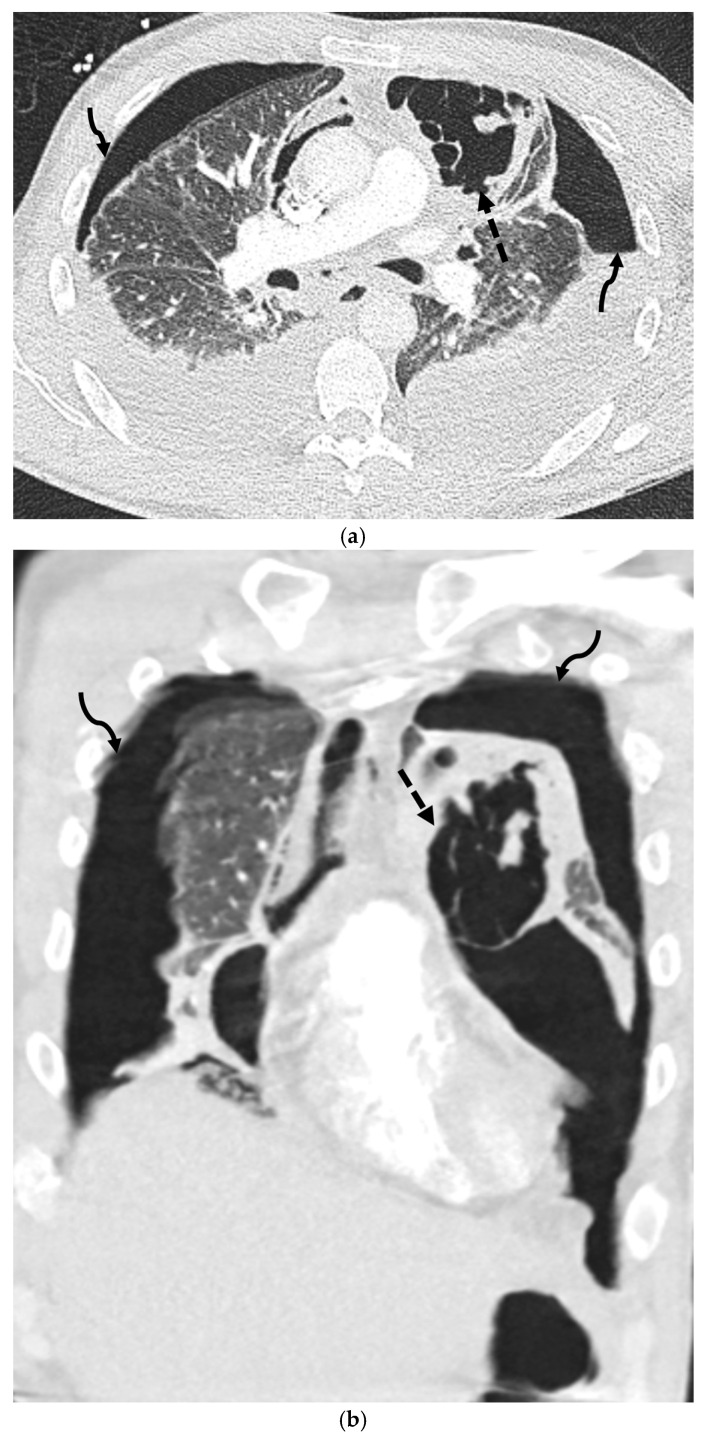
38-year-old male presenting with severe septic shock. Axial (a) and coronal (b) images from chest CT in lung windows demonstrates a cavitary lesion in the left upper lobe (dashed arrow) and bilateral hydropneumothoraces (curved arrows).
